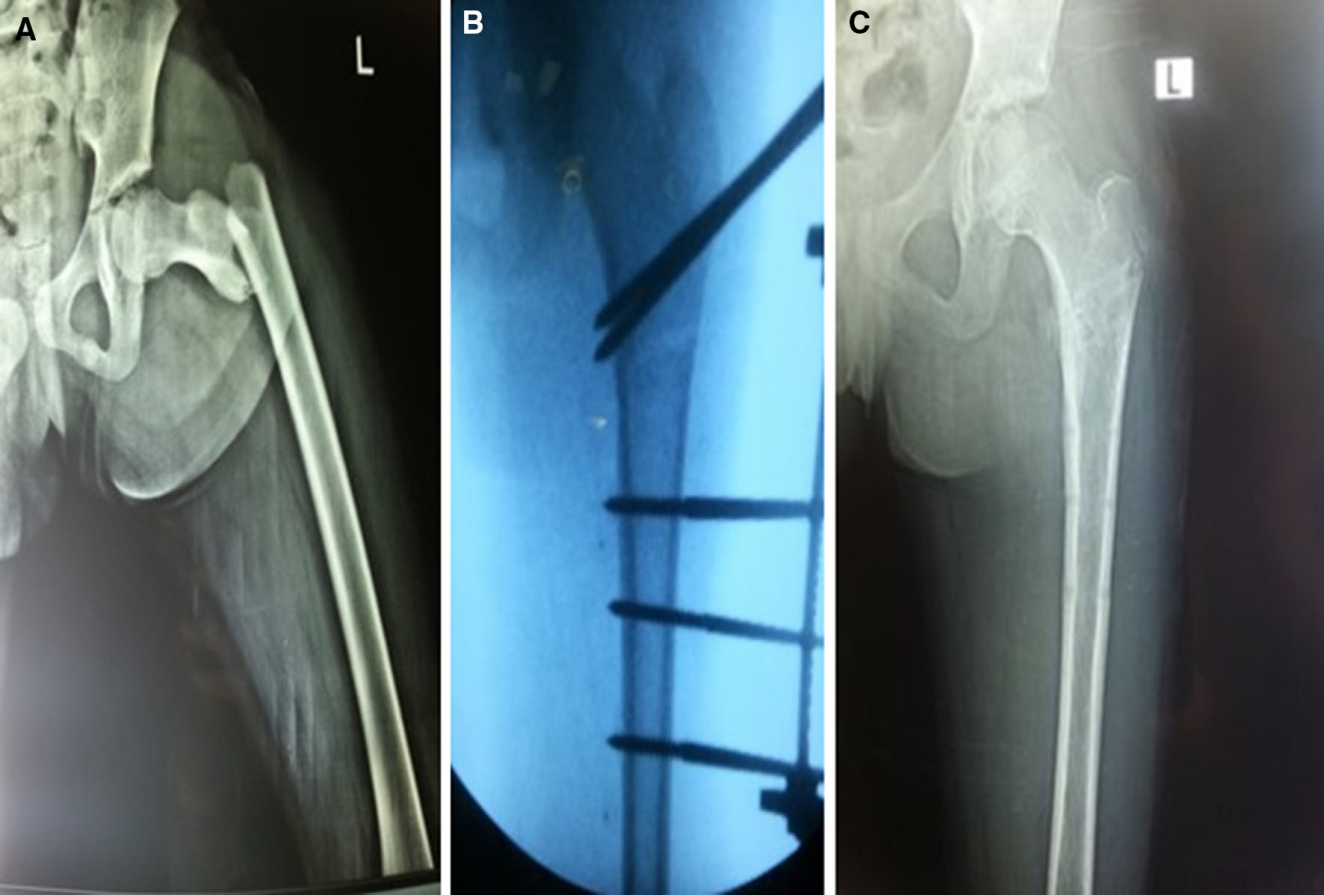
Preoperative AP X-rays (a) of 10-year-old female patient who sustained subtrochanteric fractures, intraoperative imaging after fixation (b). Anteroposterior (c) X-rays after complete union (11 weeks) and frame removal
